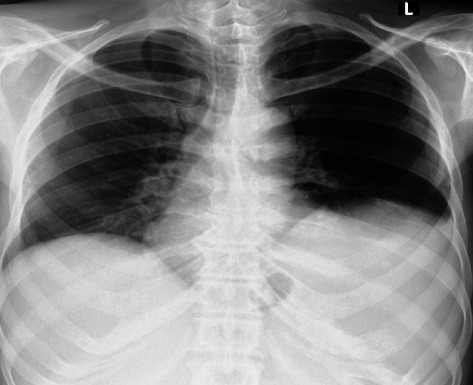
Normal PA chest X-ray with an elevated left hemidiaphragm.
